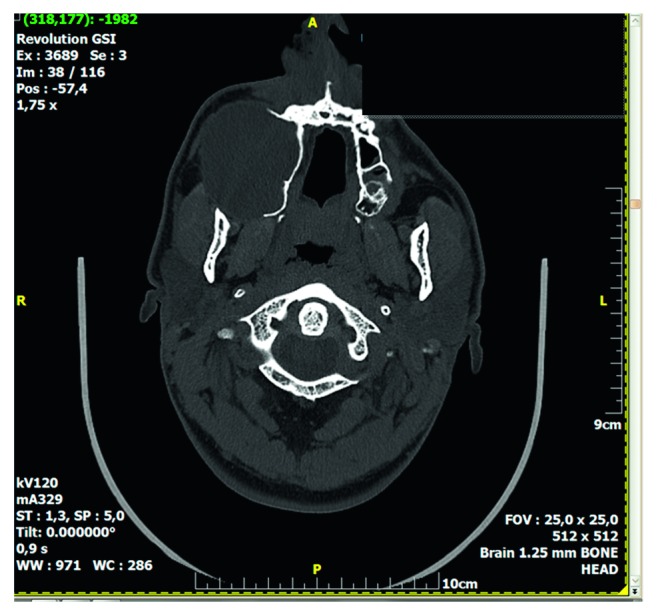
Preoperative CT, axial plane, bone window.
